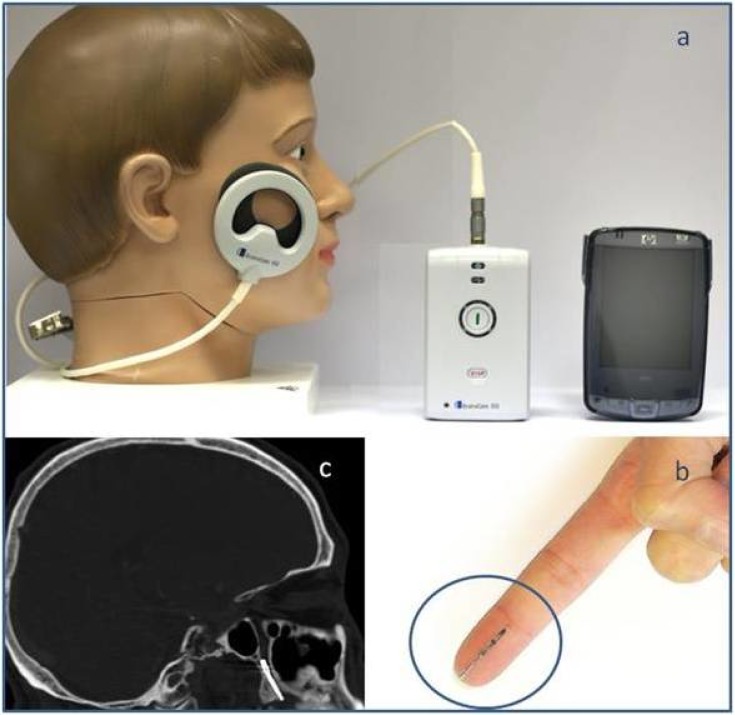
The ischemic stroke system. The energy delivery control subsystem (a) including the controller, driver and transmitter; the Implantable Neural Stimulator (b) and a sagittal view of a CT scan demonstrating the INS position (c).
